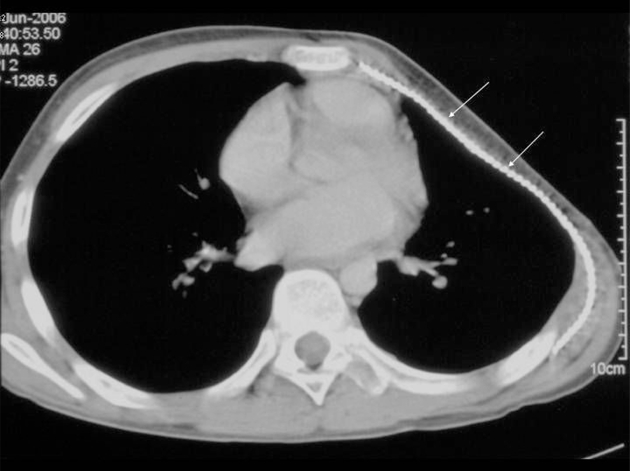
CT examination of the chest post-chemotherapy and chest wall surgery showing deformity of the left chest wall at site of extensive rib resection. The titanium mesh is seen in situ (arrows). There is no evidence of tumour recurrence.
